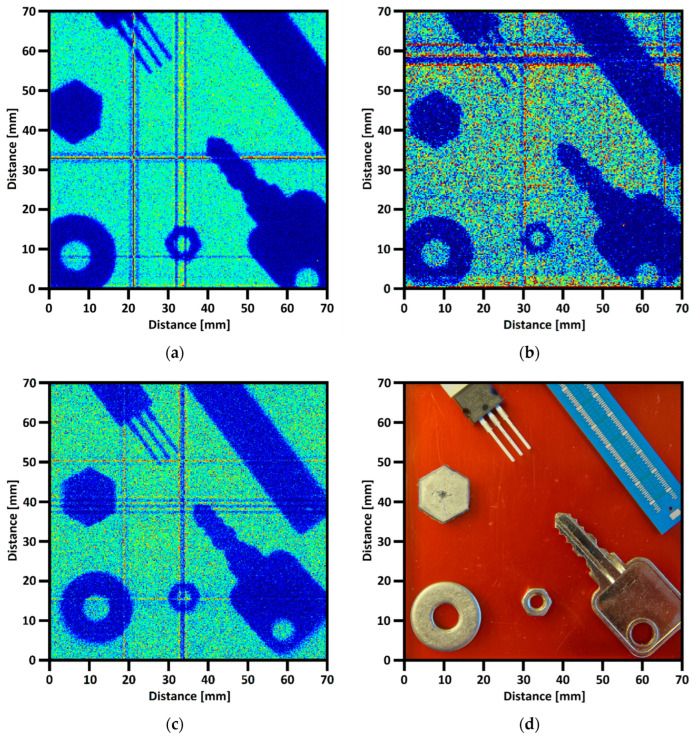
Comparison of the radiographs measured using different readout boards: (a) radiograph measured on 70 × 70 mm^2^ PCB board (128 × 127 Ch.); (b) radiograph measured on 70 × 70 mm^2^ PCB board (using only 64 × 63 Ch.); (c) radiograph measured on CERN 100 × 100 mm^2^ M.S. board [18]; (d) objects placed on the detector for the readout board tests [18].
